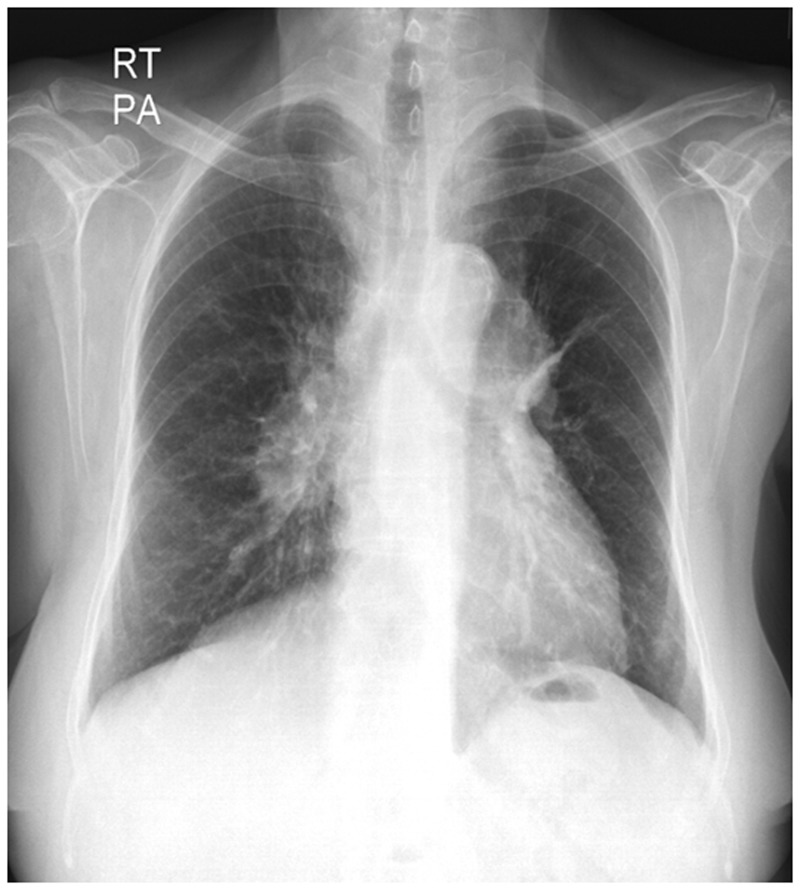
Portopulmonary hypertension. Chest radiograph of a case of portopulmonary hypertension in a patient with cirrhosis showing dilated central pulmonary arteries and pruning of peripheral pulmonary vessels suggestive of pulmonary arterial hypertension.
